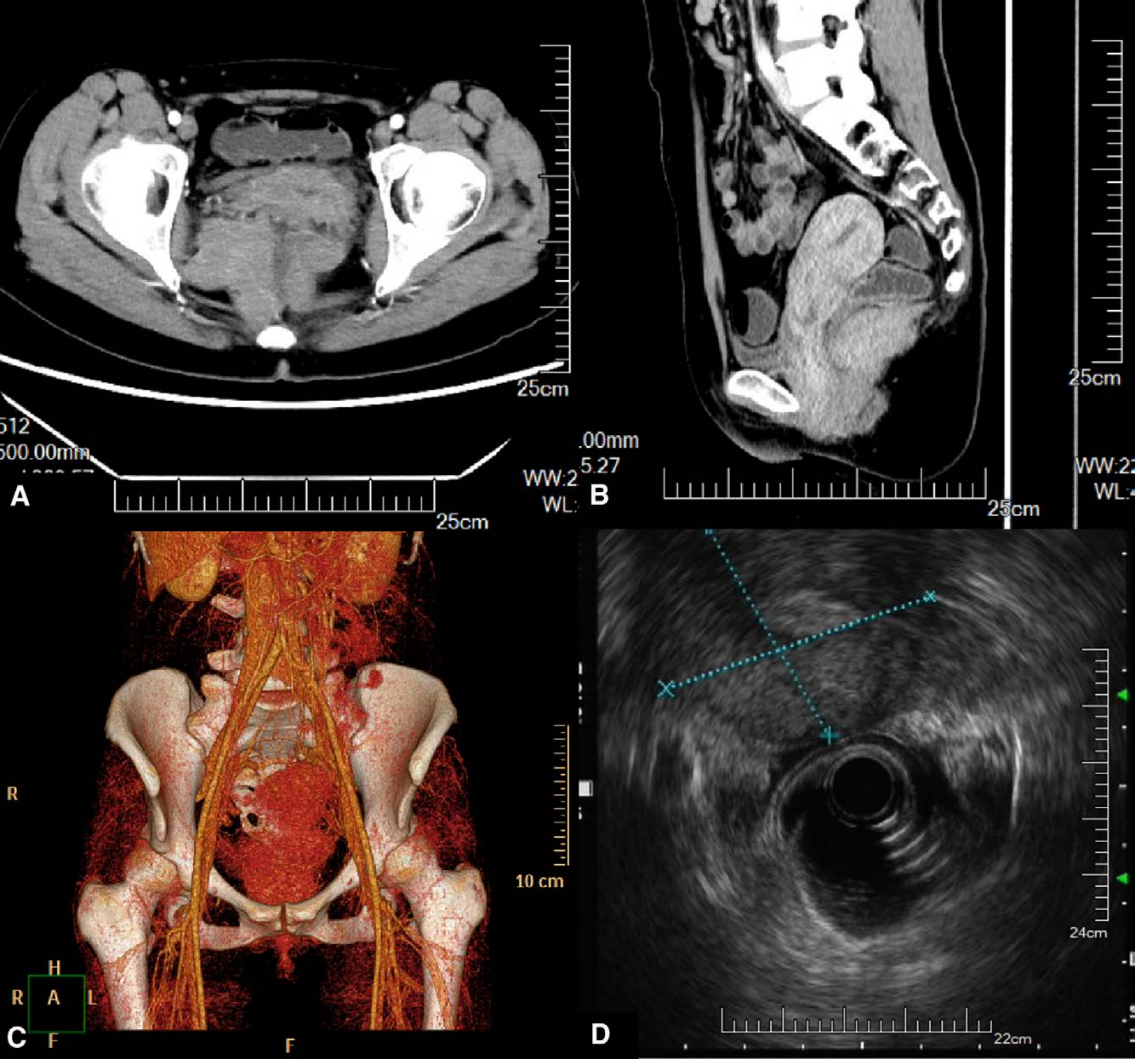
Image findings. A: Computed Tomography shows the mass very close to the levator ani muscle. B: The pararectal mass appeared to be homogeneous in density and slight enhancement in the arterial phase. C: The distal branches of bilateral internal iliac artery went into the mass. D: Endoscopic ultrasonography showed the well-defined mass with a homogeneous echotexture compressed the rectum, as well as a clear boundary to the rectal wall.
